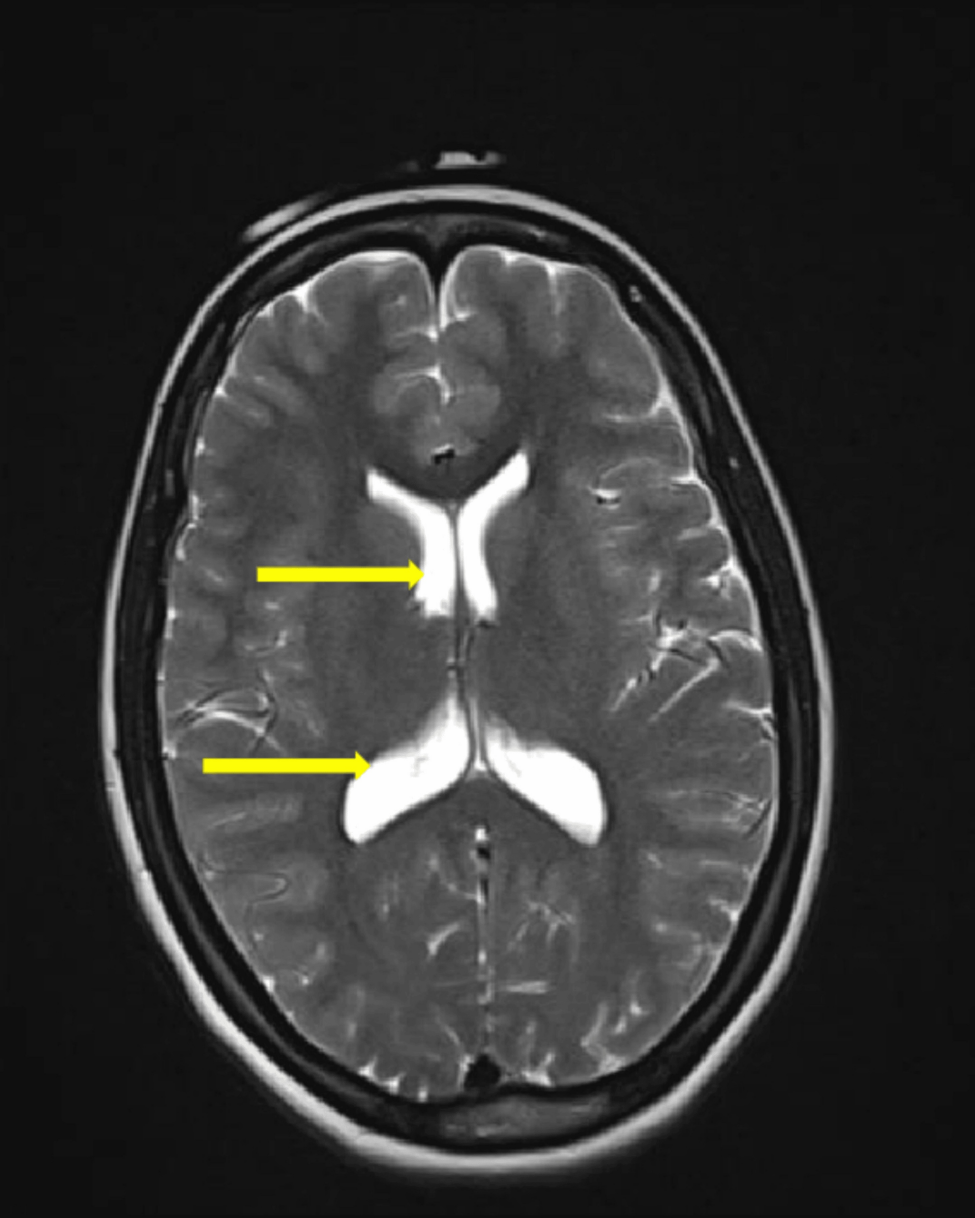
MRI brain T2 axial section shows hydrocephalus (arrows show enlarged ventricles)
